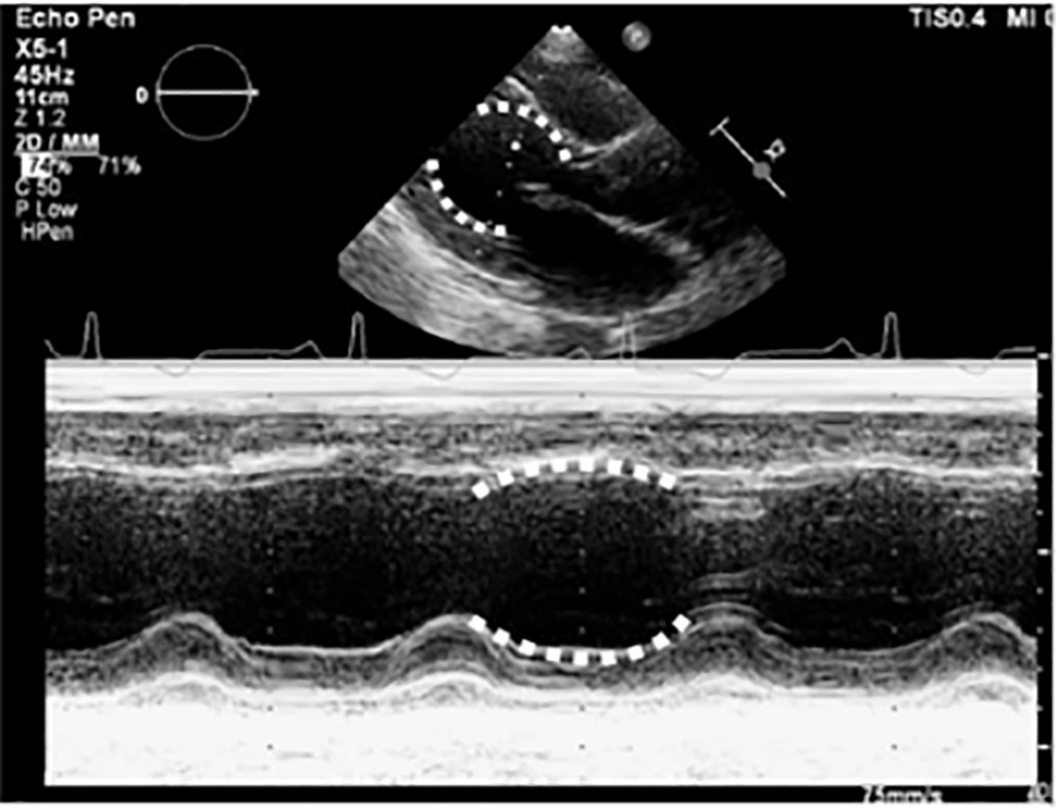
Transthoracic echocardiogram of 73-year-old female at the left sternal border showing apical ballooning (white dotted line) in the long axis (top) and m-mode (bottom).
